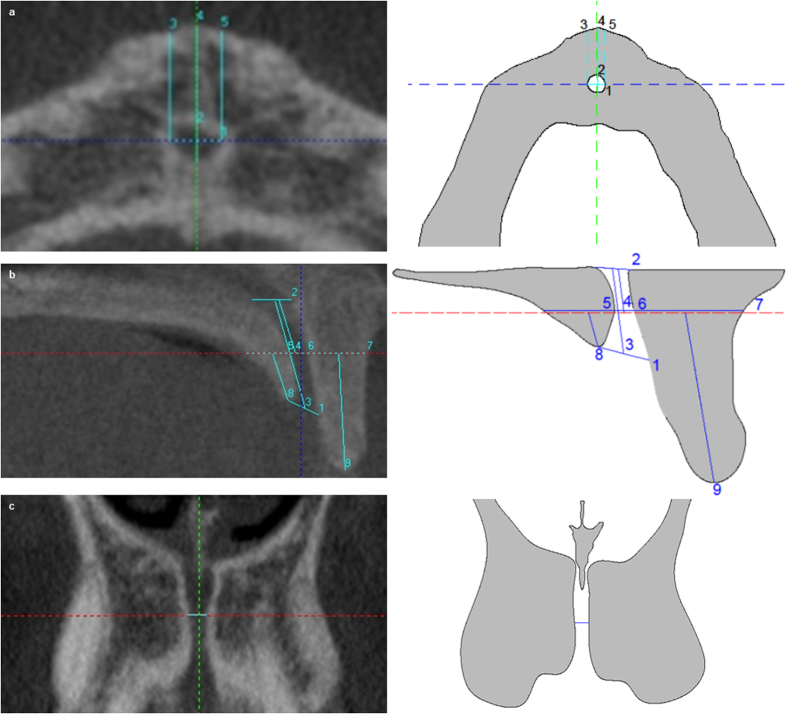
Radiographies and draw- scheme of level 2 show the follows: (a) Axial slice: (1) bpD2, (2) tD2, (3) LW2, (4) CW2 and (5) RW2. (b). Sagittal slice: (1) NC diameter in coronal region, (2) CD3, (3) NCL, (4) middle of NCL, (5) PW2, (6) SD2, (7) BW2, (8) PL2 and (9) BL2. (c) Coronal slice: CD2.
